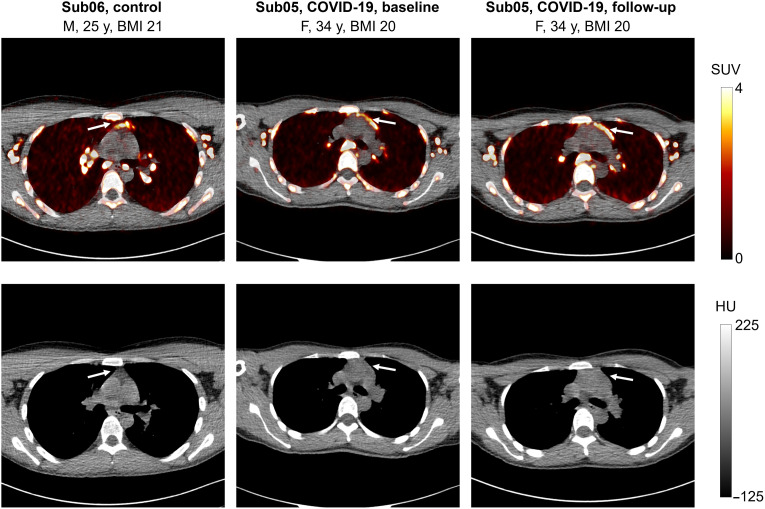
PET/CT image slices of thymus uptake. Selected transverse PET/CT image slices of one control subject and one COVID-19 convalescent patient (at baseline and follow-up scan) showing thymus uptake at 48-hour time point of imaging. HU, Hounsfield unit.
